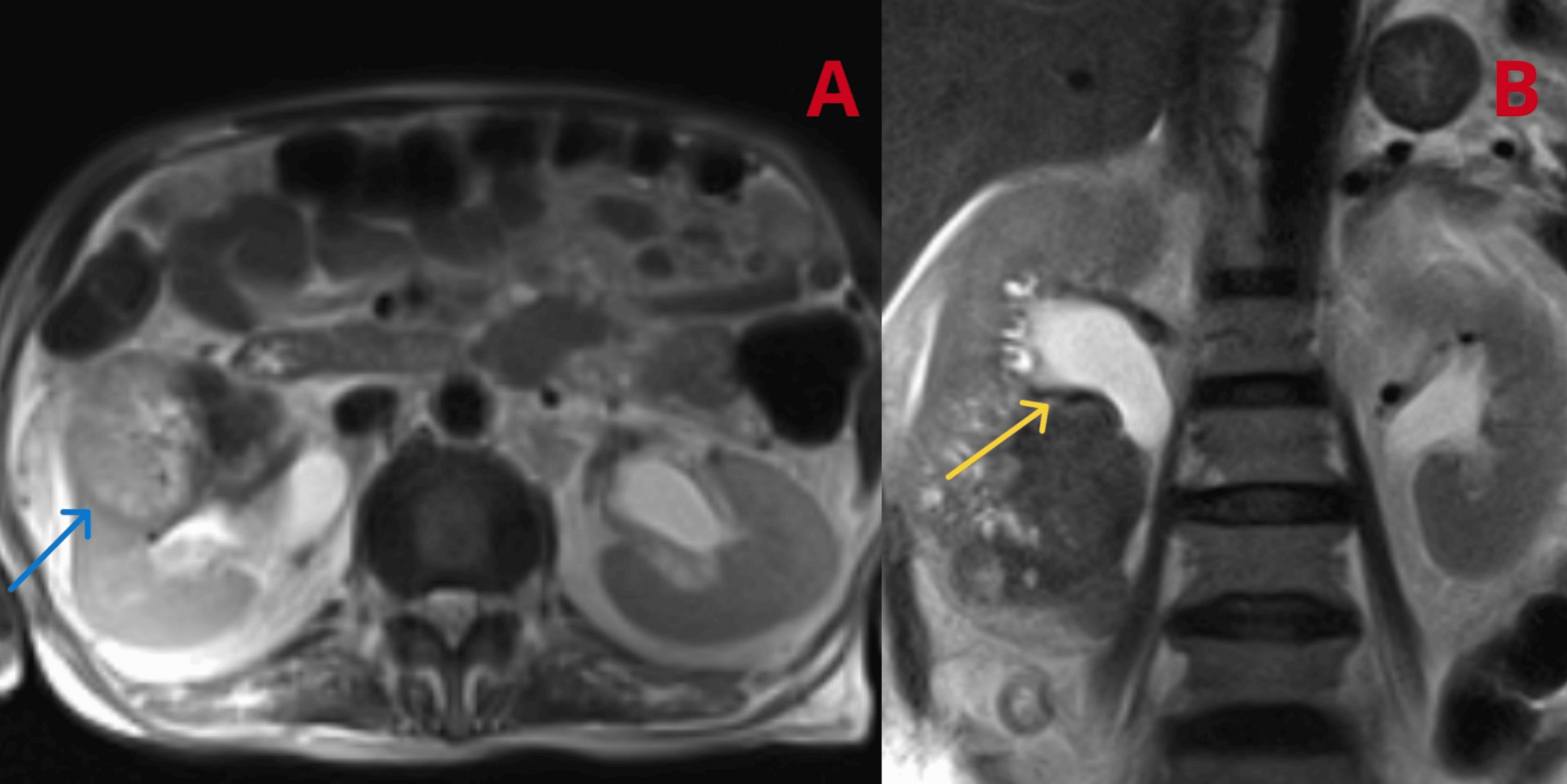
MRI abdomen and pelvis; (A) Right kidney appears enlarged and ill-defined heterogenous signal intensity lesion mid and lower pole measuring 9.6x7.1 cm (blue arrow), (B) Moderate hydroureteronephrosis noted in the right kidney (yellow arrow). The left kidney is normal in size and signal intensity. Pelvicalyceal system is not dilated.
